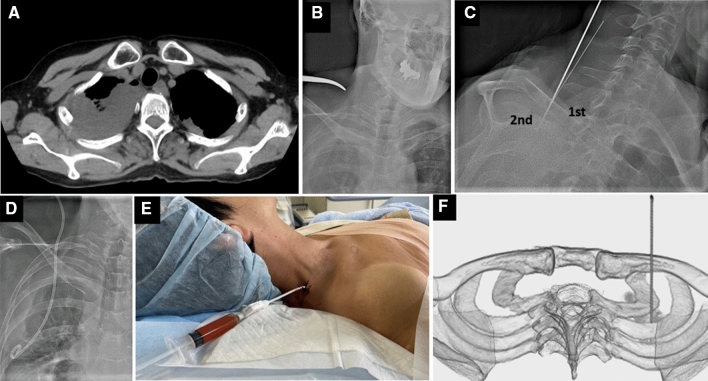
A A female patient in her sixties with right pleural effusion after a lobectomy for lung cancer. B A surgical Pean forceps pointing at the puncture site located just above the posterior first rib. C, D, E A 17-gauge needle is passed through first intercostal space with 60 ° right anterior oblique projection and an 8.5 Fr catheter (Dawson-Mueller Multipurpose Drainage Catheter; Cook, Bloomington, IN, USA) was inserted. F A 3D-CT showing the needle trajectory
